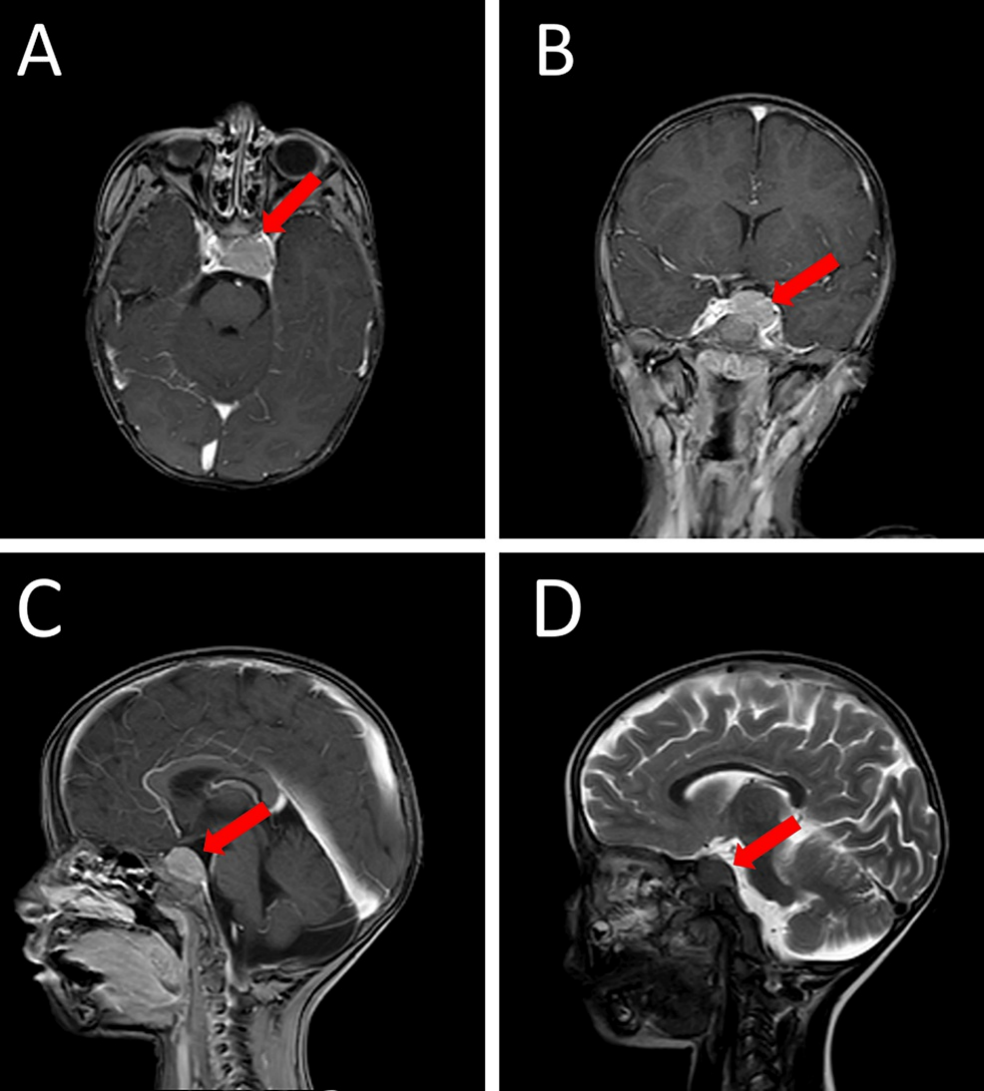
Preoperative MRI of sellar solid lesion (red arrows) extending to the left cavernous sinus, and postoperatively diagnosed as xanthogranuloma of the sellar region. The lesion shows isointense signal in T1WI and hypointense signal in T2WI (D) with homogenous enhancement with gadolinium (A: axial, B: coronal and C: sagittal). The tumor compressed the pituitary gland and the optic chiasma upward. MRI = magnetic resonance imaging, T1WI = T1-weighted images, T2WI = T2-weighted images
